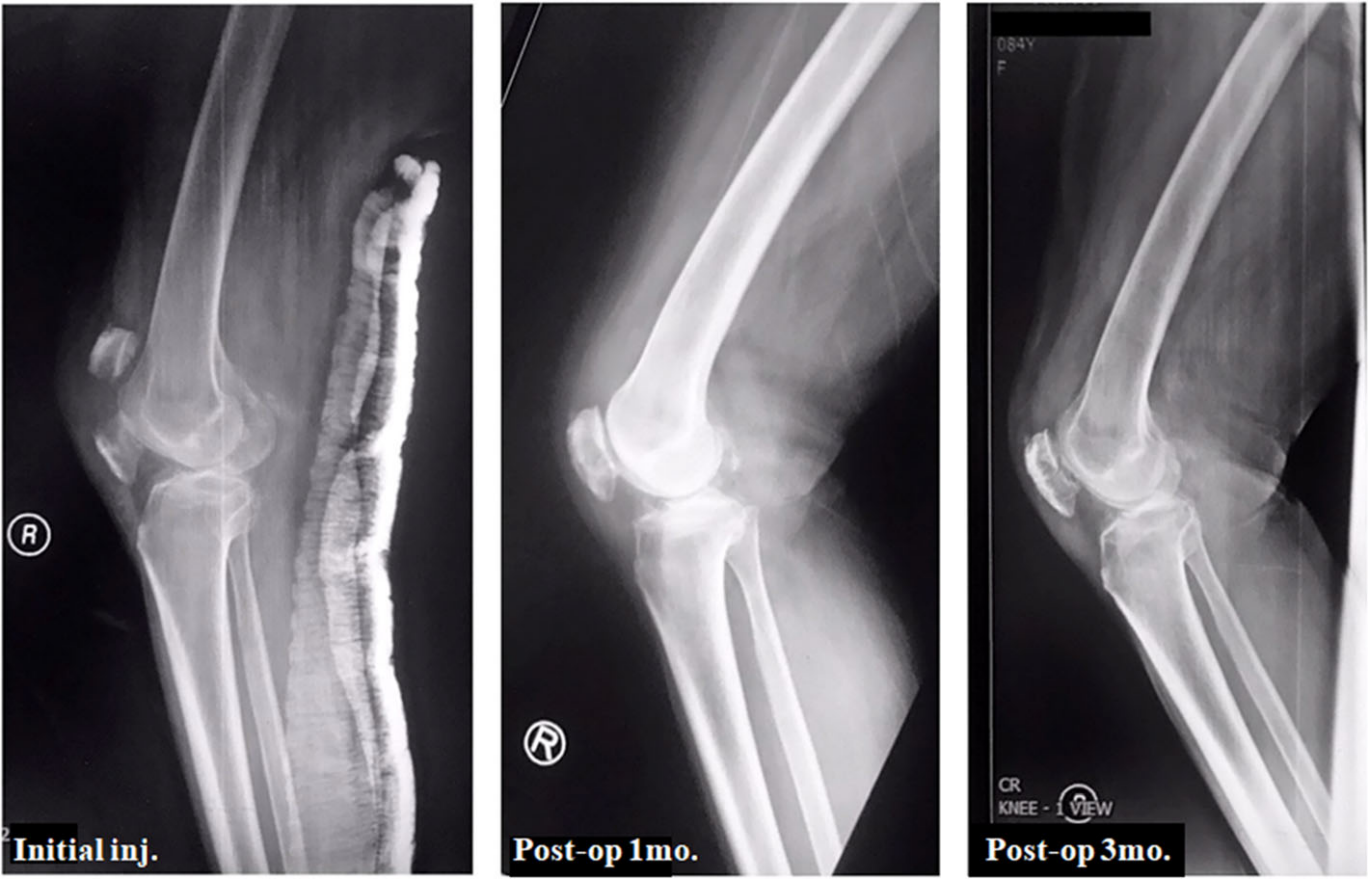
An 84-year-old female with underlying primary osteoarthritis and postmenopausal osteoporosis experiencing direct injury to the right patella. The initial radiograph reveals an articular displaced fracture of the right patella. A bone union is seen in 3 months postoperative radiograph without complications
